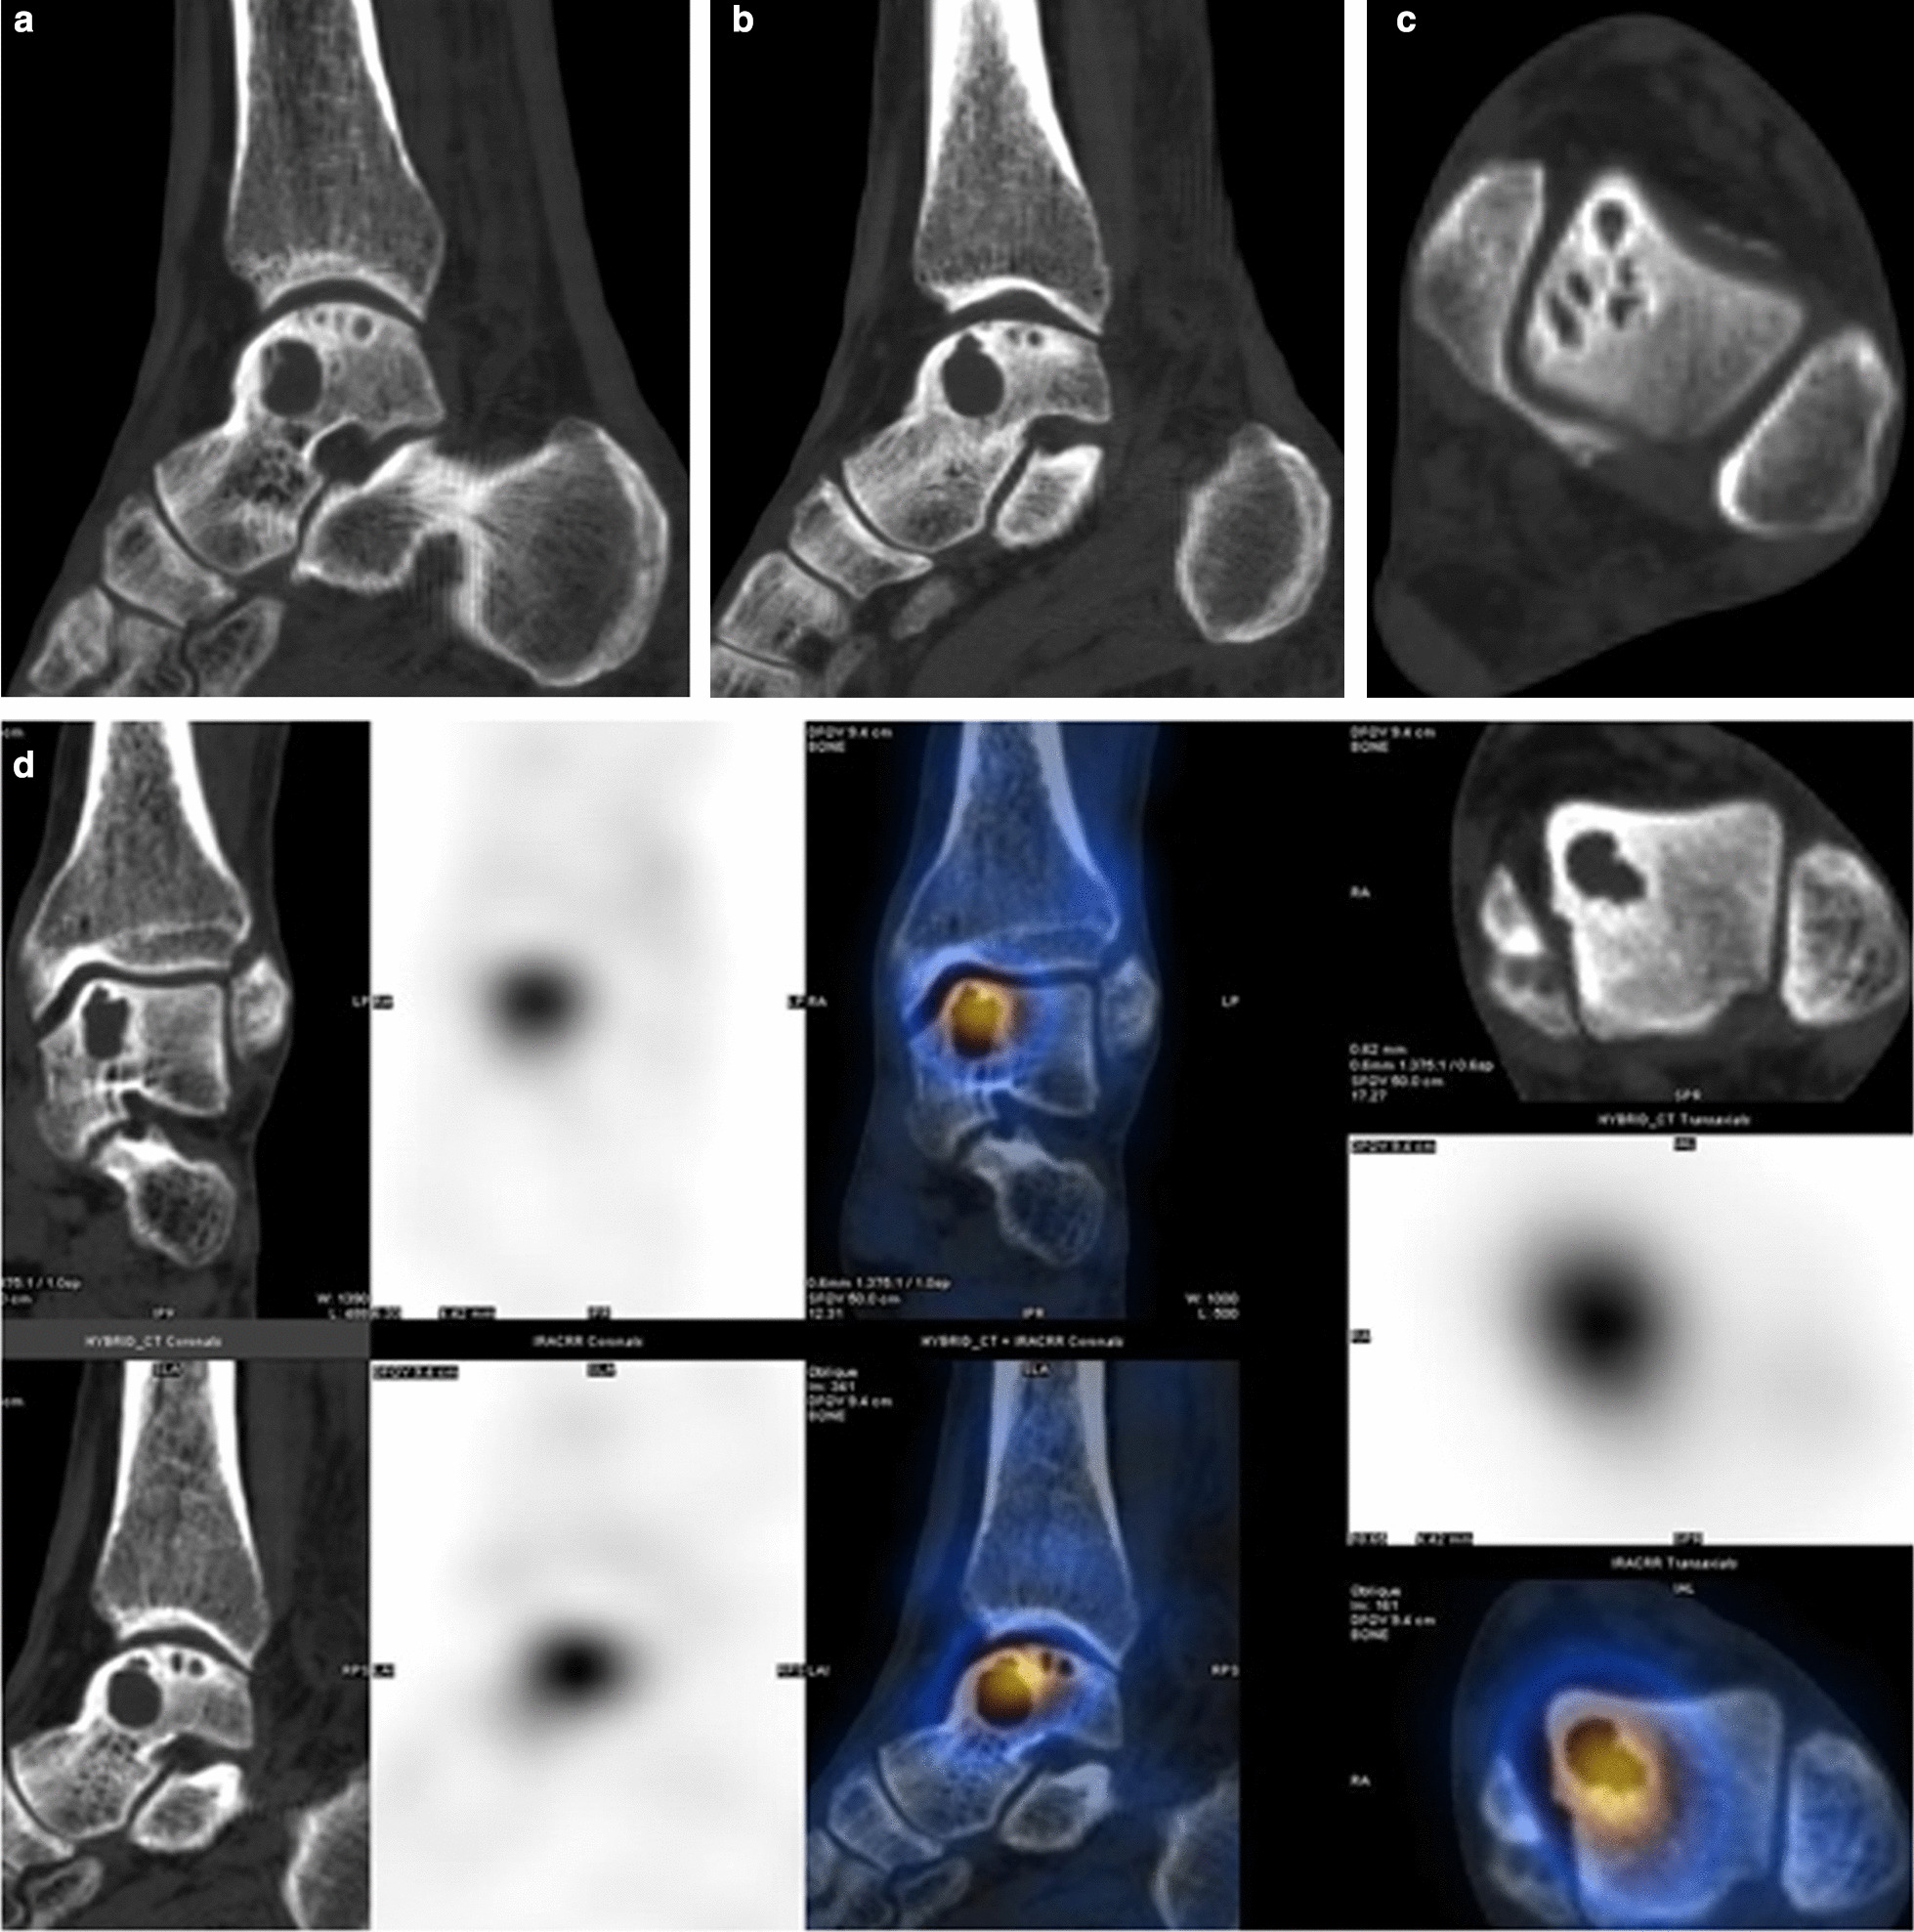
A 65-year-old man who had left ankle pain for 4.5 years that was then aggravated for 1 week. The fusion image shows that the lateral fornix of the left talus was locally fractured and the articular surface was not smooth. Multiple small capsular hypodense shadows can be seen underneath it, with obvious sclerosis of the edges. A larger cystic hypointense shadow can be seen below the anterior part of the small cyst, and a narrow channel can be seen connecting with the articular surface. The local bone cortex was not continuous. The area of anomalous tracer concentration in the left talus corresponds to the area of foraminal cystic degeneration (Type II cystic lesion). a Sagittal type II large cyst formation; b large cyst communicating with the articular surface; c multiple small cysts in an axial position; d SPECT/CT fusion image
